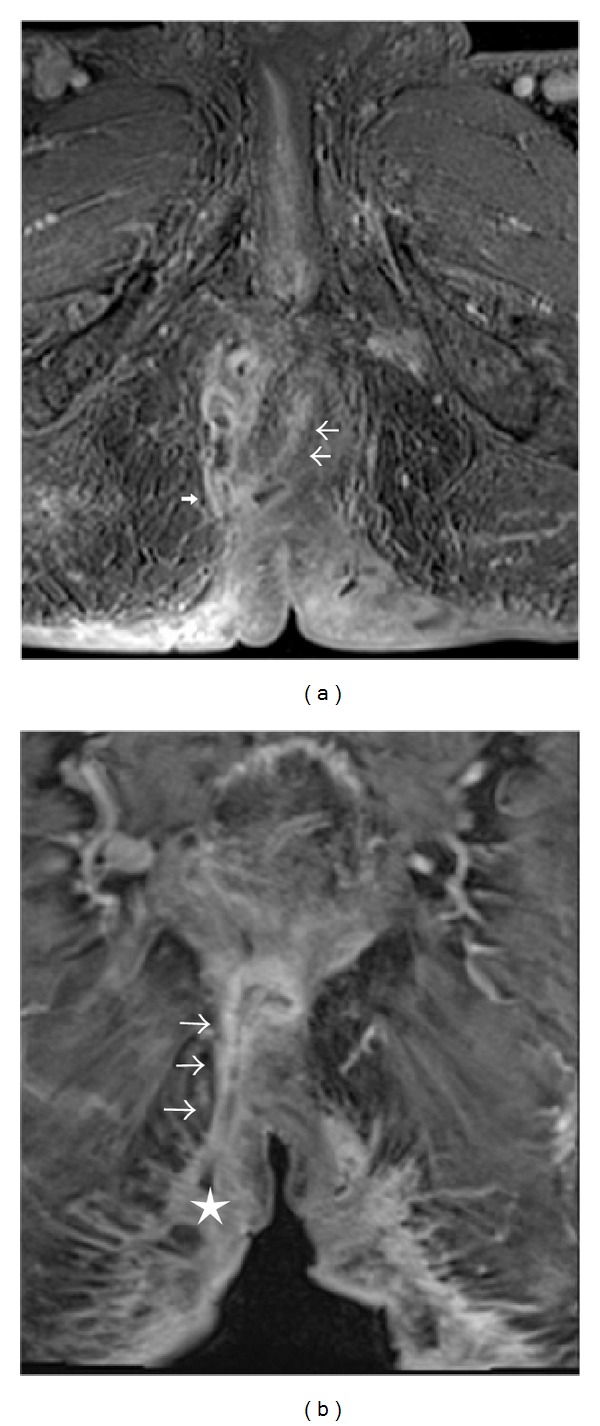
Axial T1-weighted fat-suppressed axial (a) and coronal (b) images postcontrast in an MRI pelvis done 3 years before presentation using fistula-in-ano protocol demonstrates an enhancing transphincteric fistula tract at 6 o'clock position (white arrows in both images) extending to the right ischioanal space (thick arrow) with cutaneous opening at the right gluteal region (star).
